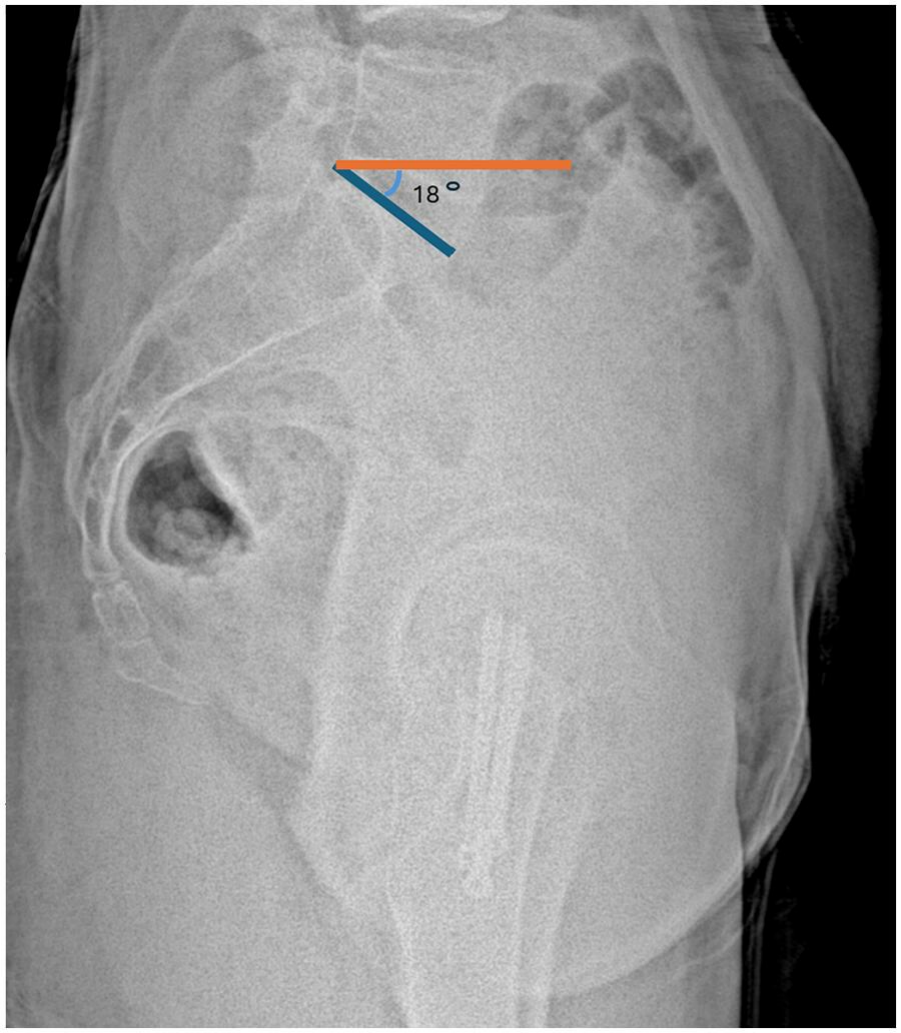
Measurement of sacral slope difference (ΔSS). ΔSS represents the change in sacral slope between standing and sitting lateral lumbosacral radiographs.
